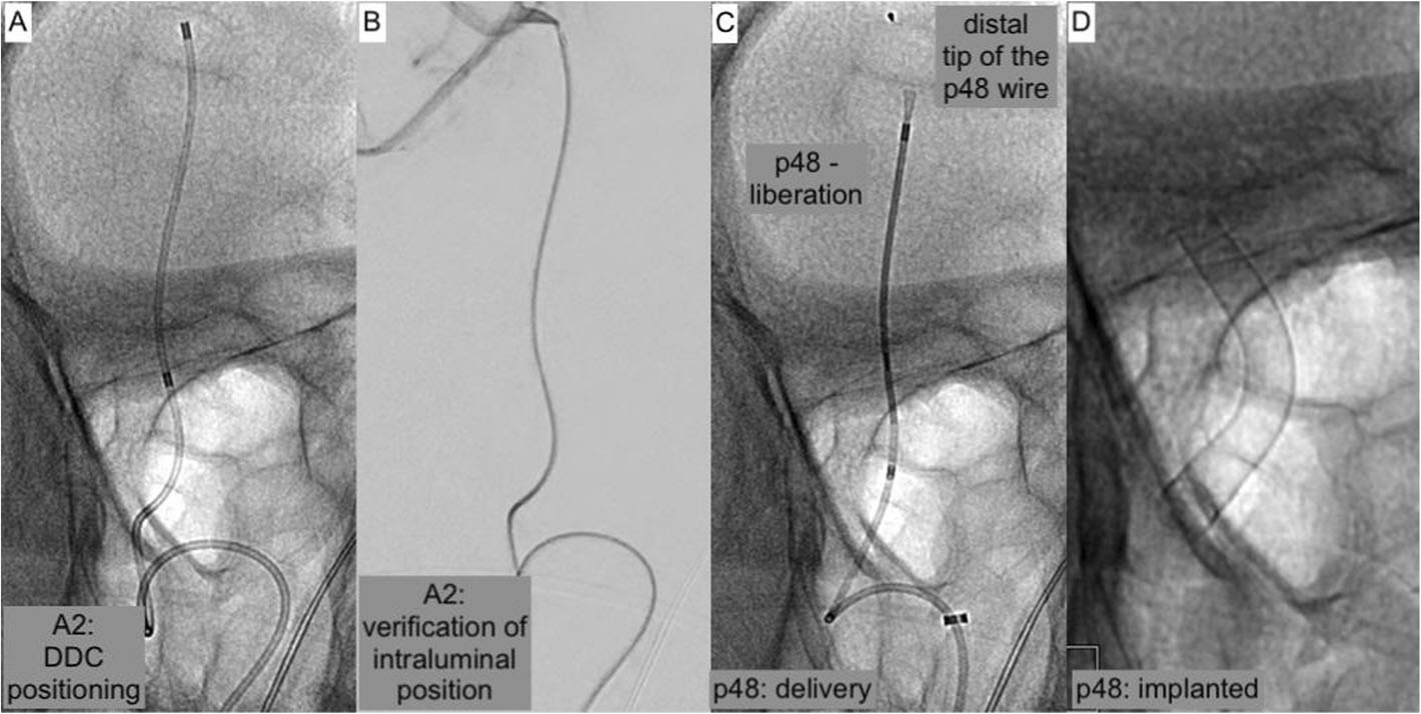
demonstrates the second step of the maneuver – introduction and placement of the greater and potentially more traumatic large bore microcatheter and the succeeding FDS implantation. a Plain radiography in working projection shows the placement of the delivery-microcatheter in the distal A2 segment, providing sufficient forerun for the delivery of the densely woven FDS. b DSA confirmation of the true lumen by injection of contrast agent via the correctly positioned microcatheter prior implantation. c Plain radiography in working projection demonstrating FDS delivery – note the radiopaque olive at the distal end of the p48 wire and the tulip-like appearance of the partially unfolded distal device. d Plain radiography of the implanted FDS, optimally covering the aneurysm neck originating from the A1-A2 curve
